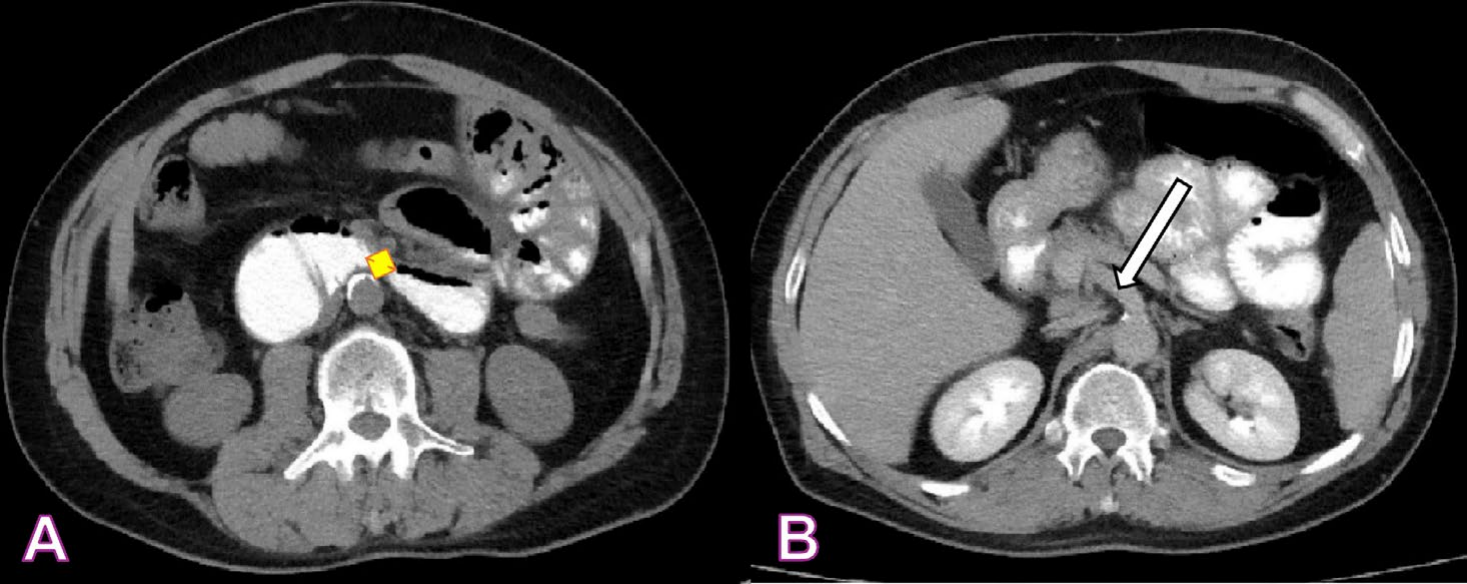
Contrast‐enhanced computed tomography (axial plane). (A) Aortomesenteric distance exceeding 12 mm; (B) Superior Mesenteric Artery indicated by a white arrow.
